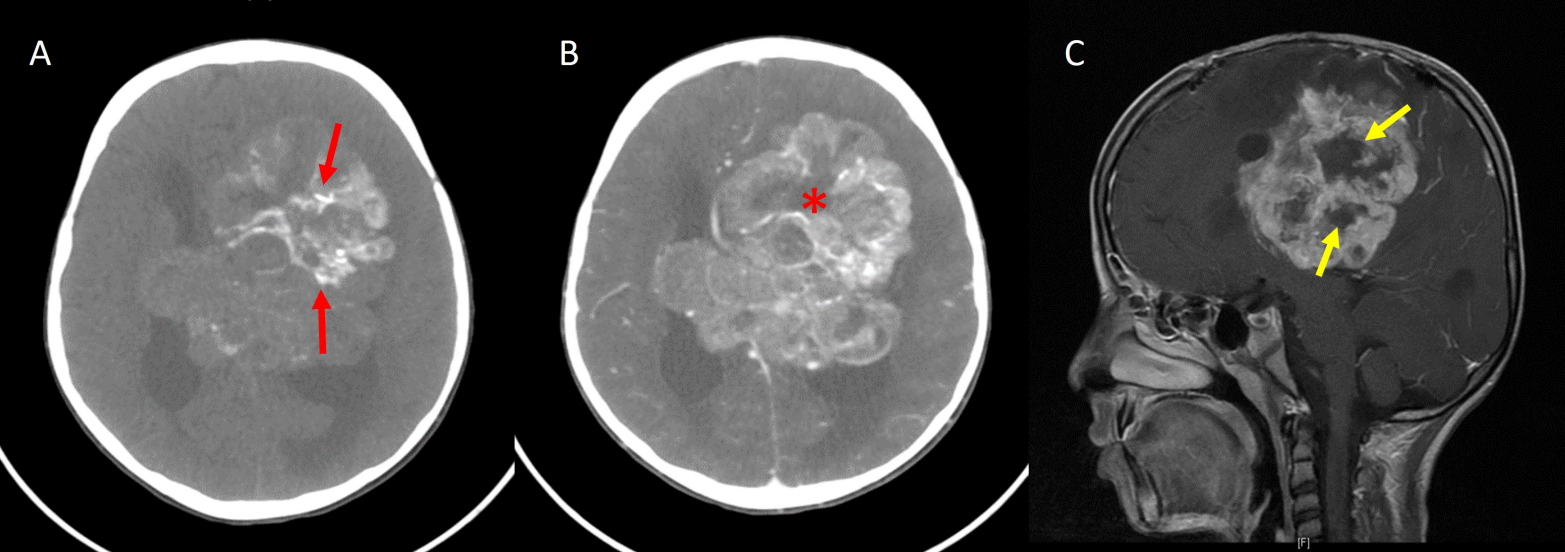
Periwinkle sign. A. Axial noncontrast-enhanced CT image shows a large heterogeneous mass in the periventricular location of the left frontoparietal lobe with centripetal calcification (red arrows); B. axial contrast-enhanced CT image showing heterogeneous enhancement of the lesion with the central necrotic area (asterisk), resembling the shape of a flower; C. sagittal contrast-enhanced T1W image of the same patient also shows a central non-enhancing area of necrosis in the shape of a flower (yellow arrows)
